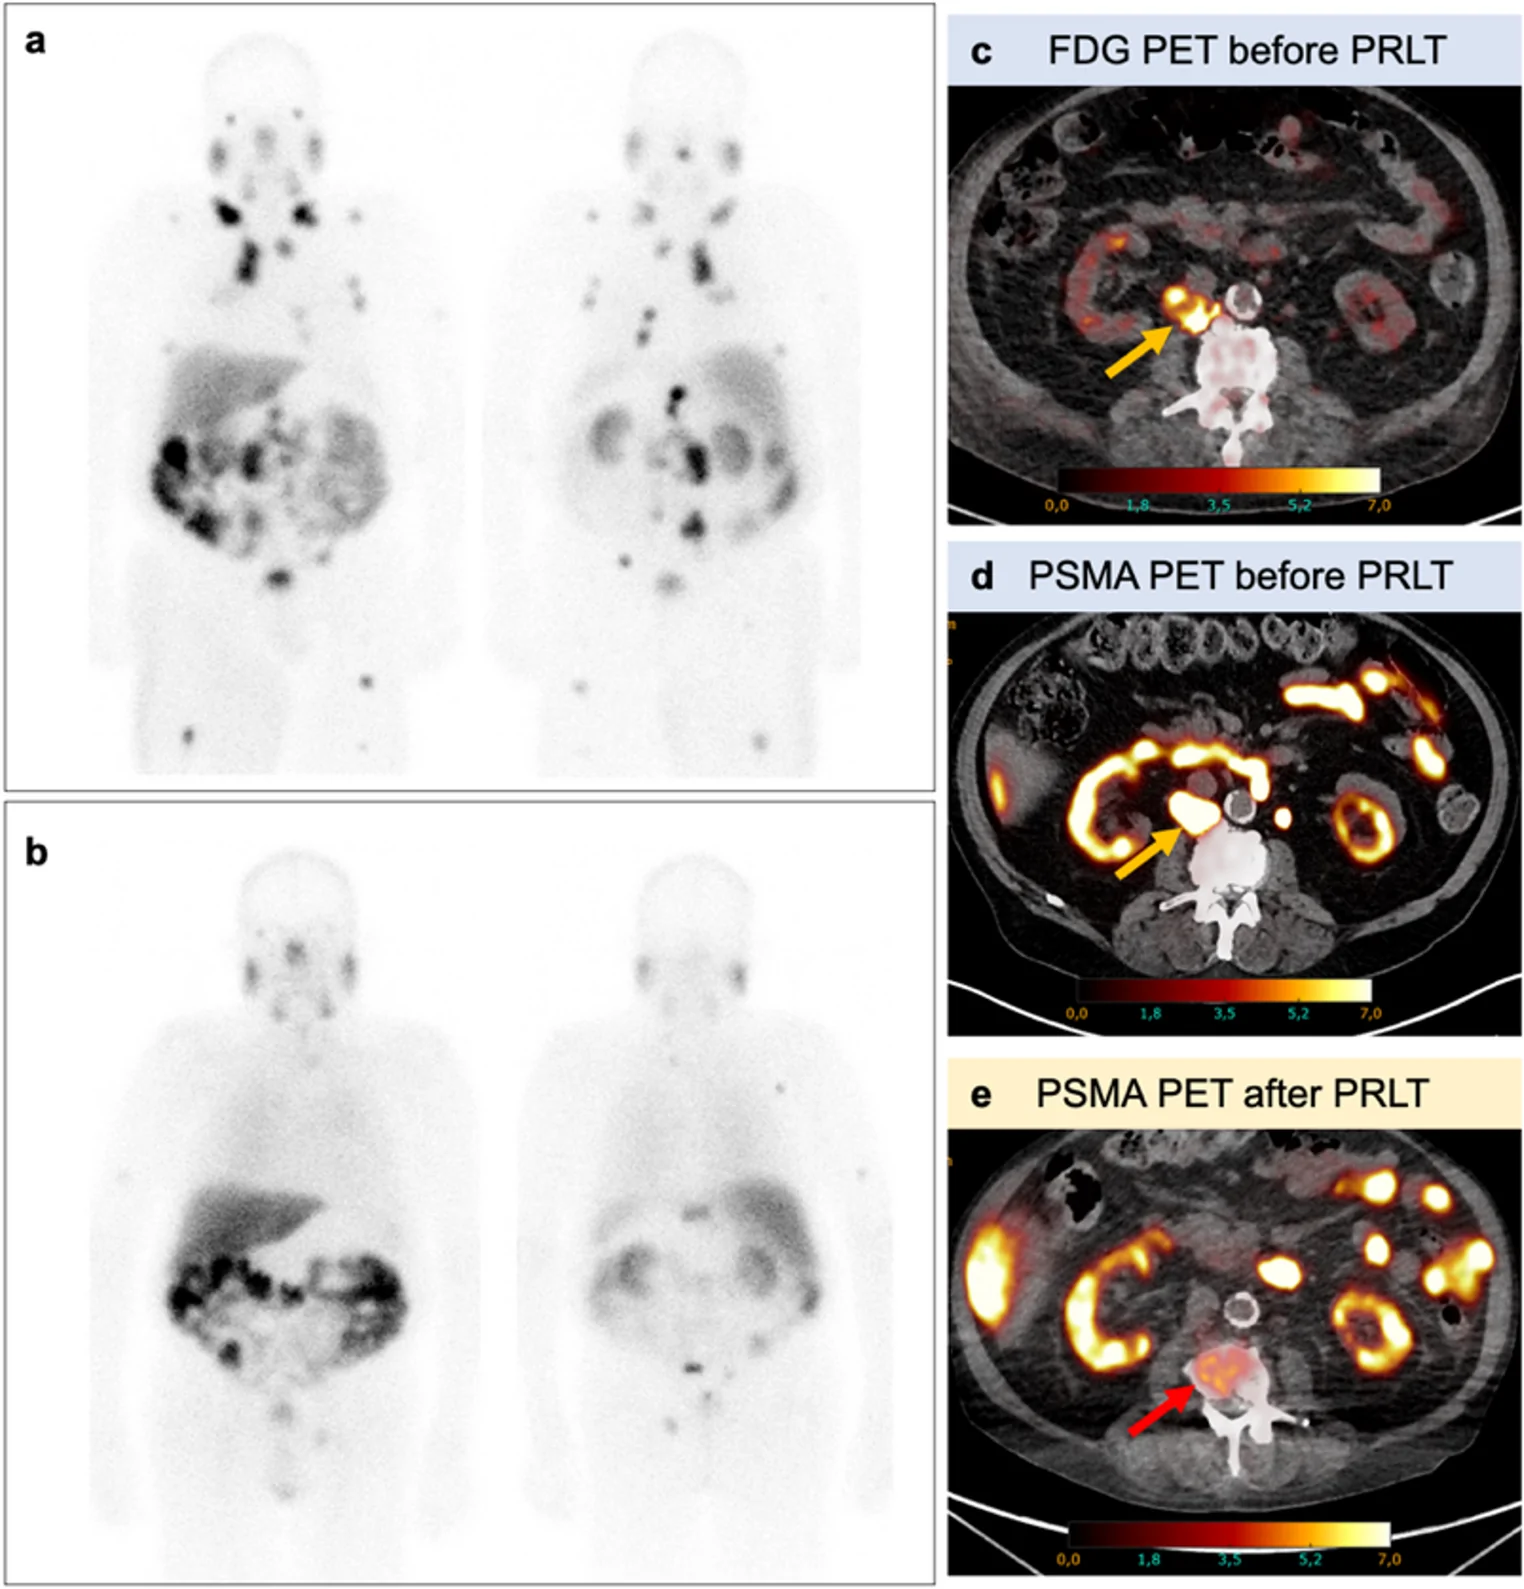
Mixed response to [^177^Lu]Lu-PSMA-I&T. Post-treatment [^177^Lu]Lu-PSMA-I&T scan showed a clear decrease of PSMA uptake from the 1 st (a) to the 4th (b) treatment cycle of the known sites of metastases, as well as the appearance of new lesions. Pre-treatment dual tracer PET/CTs demonstrate extensive metastatic disease involving lymph nodes (c-d, yellow arrows) and bone. Post-treatment [^68^Ga]Ga-PSMA-11 PET reveals an overall reduction in total disease burden, yet with new active bone lesions (e, red arrow), consistent with a mixed response. PSA levels decreased from 8.61 ng/mL before PRLT to 0.894 ng/mL, corresponding to a percentage reduction of 90%
